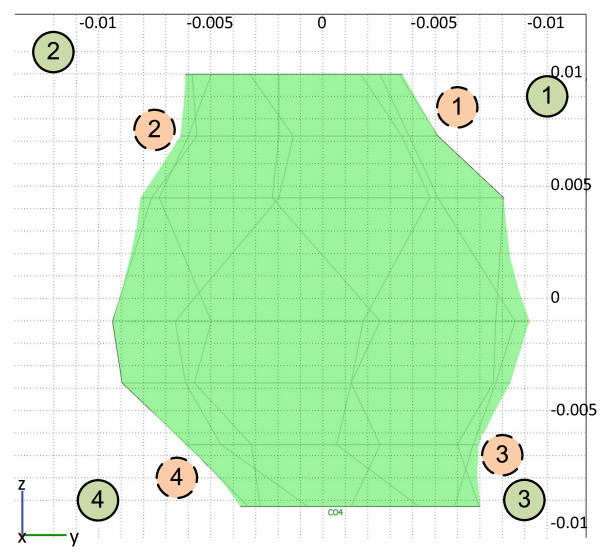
Actual positioning of the electrodes relative to the treatment plan. Electrode positions according to the treatment plan (dashed line circles) and actual electrode positions during treatment as determined by ultrasonography and photo documentation (solid line circles). The presented tumor cross-section is a parallel projection of the tumor on a plane parallel to the skin surface.
